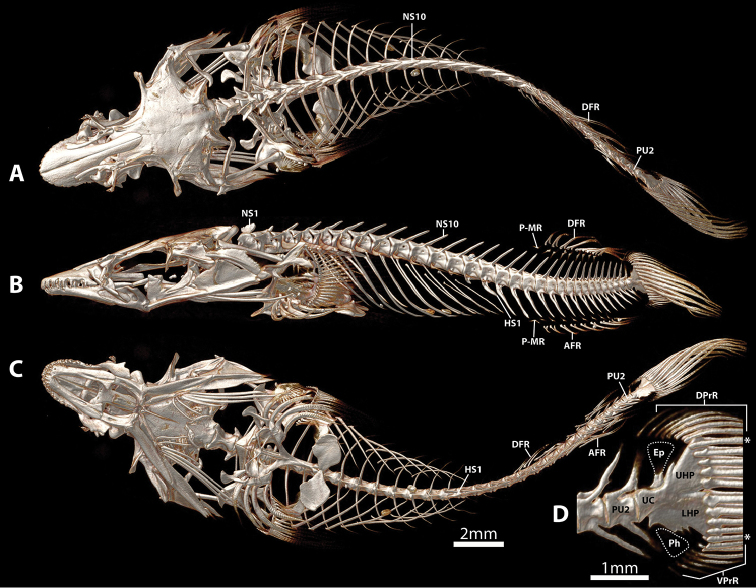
CT scanned skeleton of *Dellichthys
